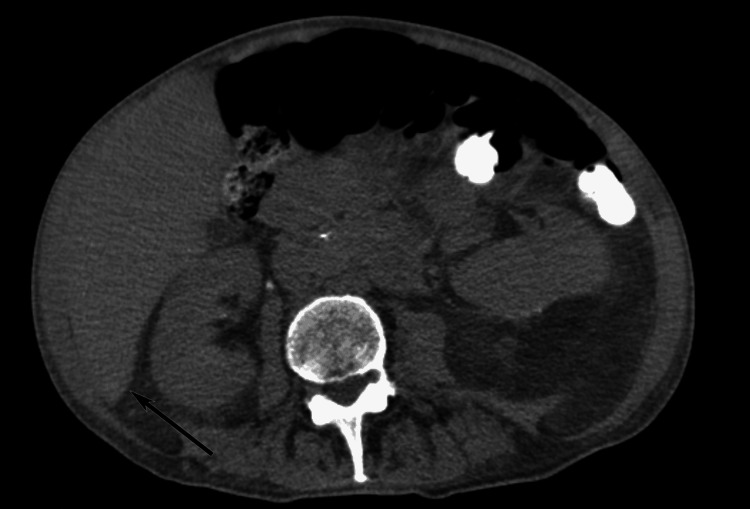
Riedel's Lobe Abdominal computed tomography (CT) scan without intravenous contrast medium. The Riedel’s lobe appearing as a tongue-like projection of the right lobe’s inferior border of the liver is highlighted (black arrow).
